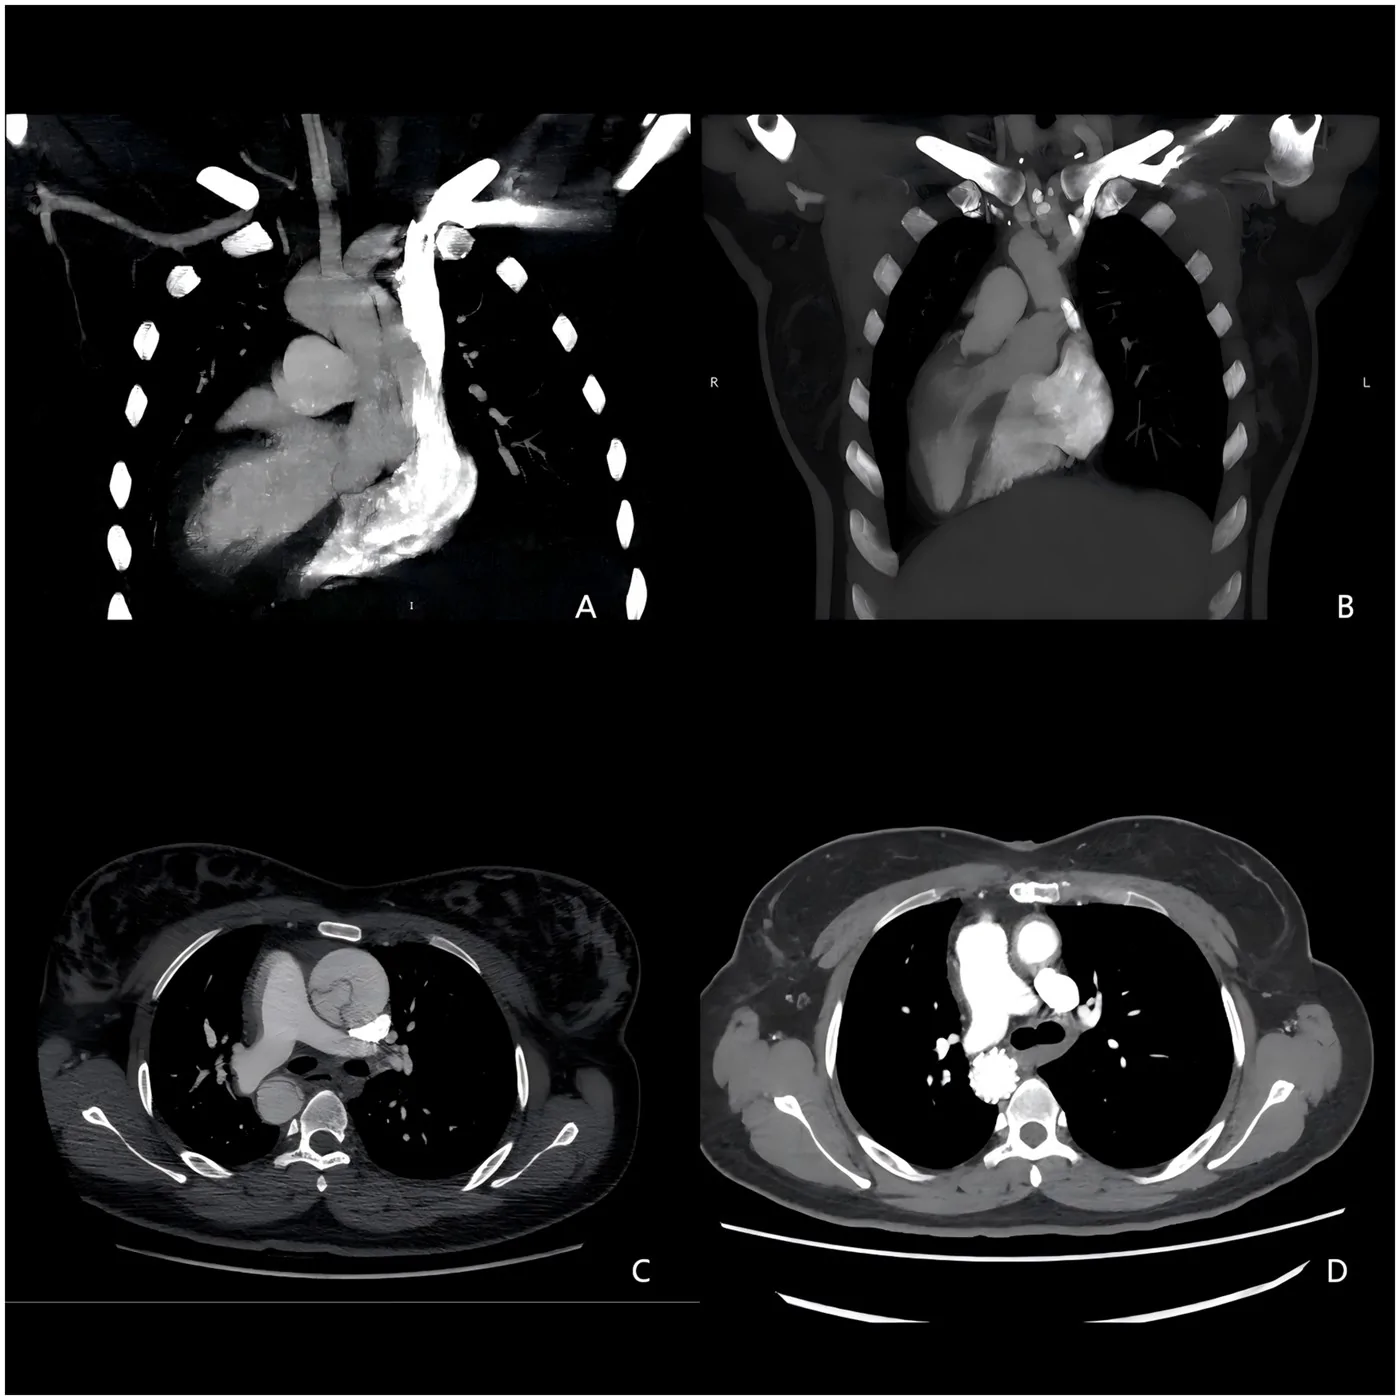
Preoperative and Postoperative Computed Tomography Angiography (CTA). (A) Preoperative coronal image showing supra-aortic dissection and nonopacification of the distal left brachiocephalic artery. (B) Postoperative coronal image showing patent reconstructed supra-aortic branches. (C) Preoperative axial image showing an ascending aortic intimal flap. (D) Ten-year follow-up axial image showing thrombosis of the false lumen around the stent-graft.
